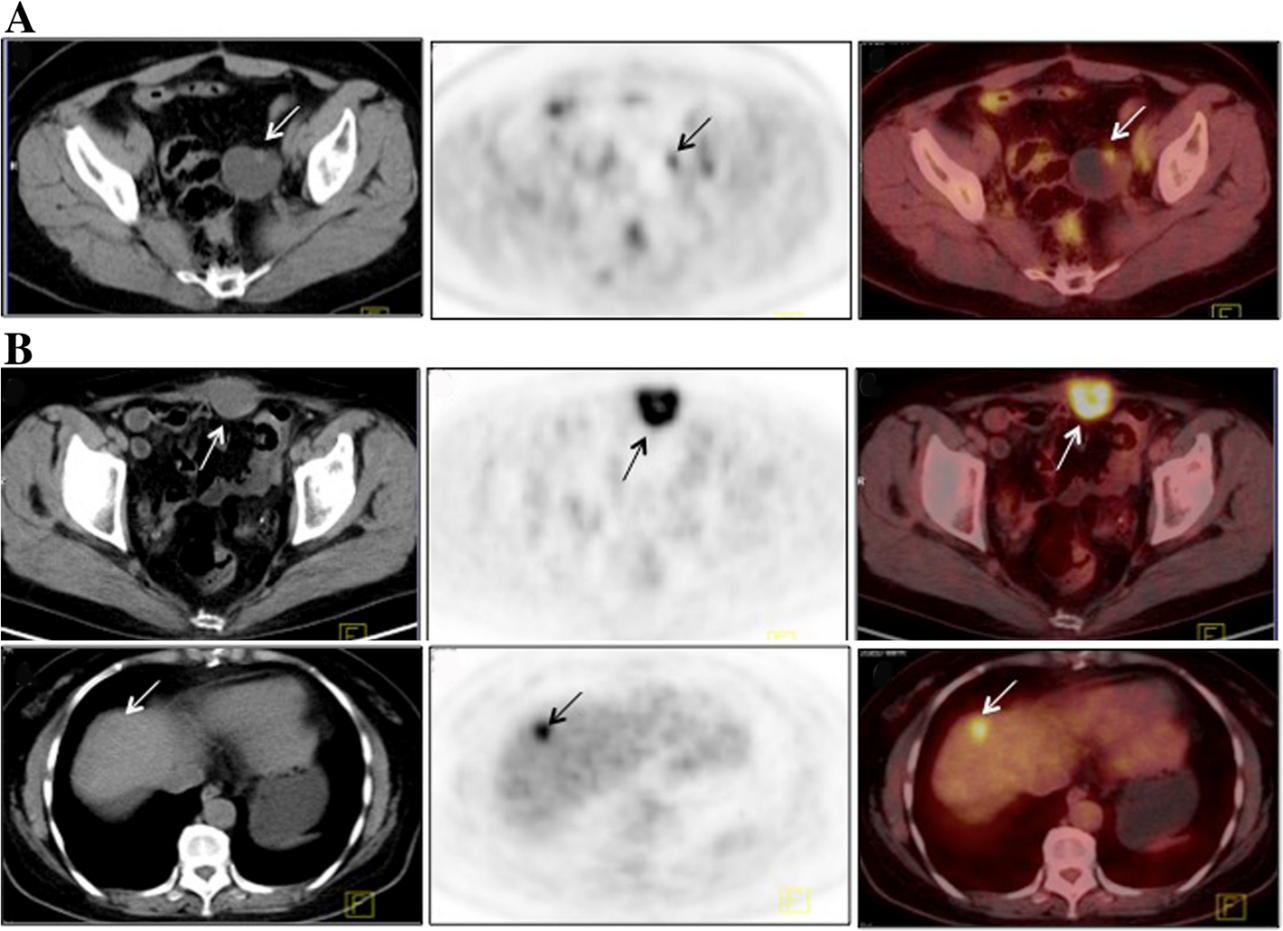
Representative PET/CT images. a:The patient had vaginal cuff recurrence. The SUV max, MTV60 and TLG60 was 4.1, 0.98 mL and 3. 19 g, respectively. The overall survival (OS) and post-relapse survival (PRS) was 41 and 39 months, respectively. b:In another patient, the recurrent tumors were located in the abdominal wall and liver. The SUV max, MTV60 and TLG60 was 13.4, 9.03 mL and 82.76g, respectively. The OS and PRS was 12 and 6 months, respectively. Abbreviations: MTV: metabolic tumor volume; TLG: Total lesion glycolysis
